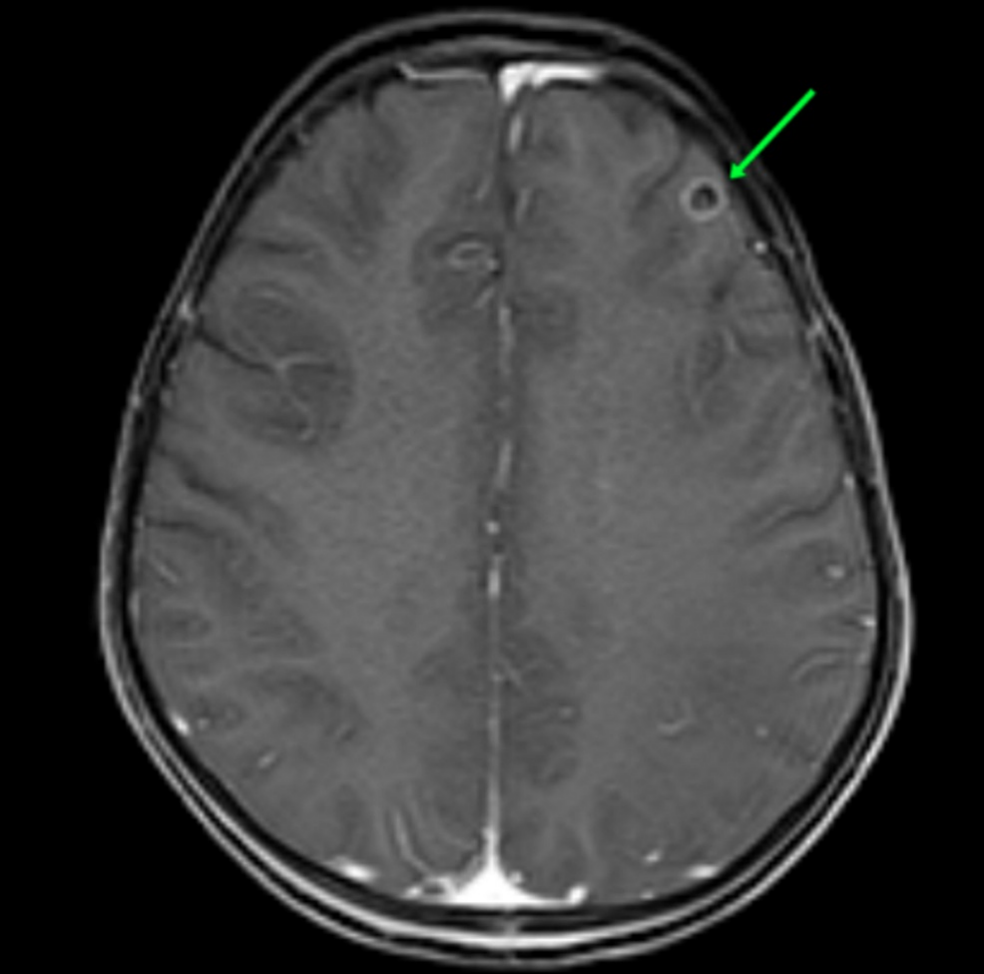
Axial T1-weighted post-contrast image Incidental finding of a small peripherally enhancing neurocysticercosis lesion in left frontal lobe (green arrow).
